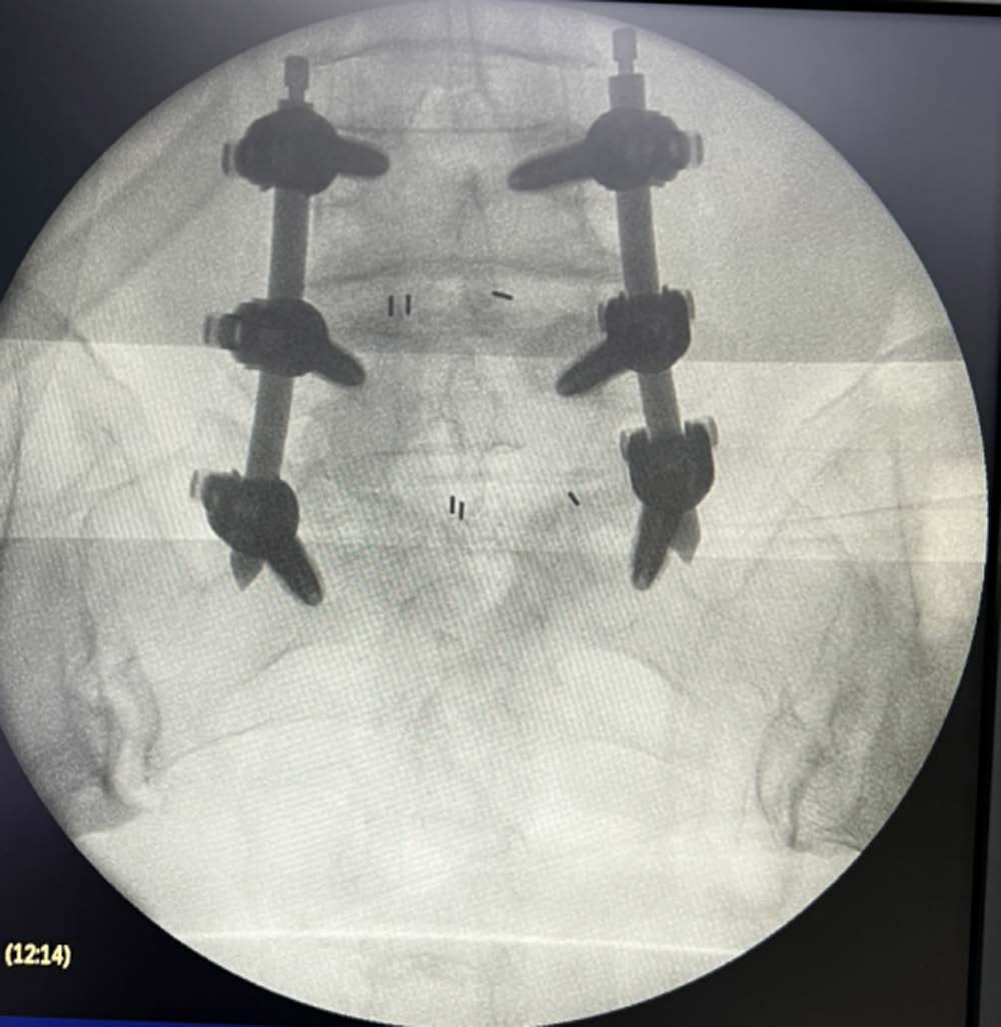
Spine radiograph with anteroposterior view during the operation.
